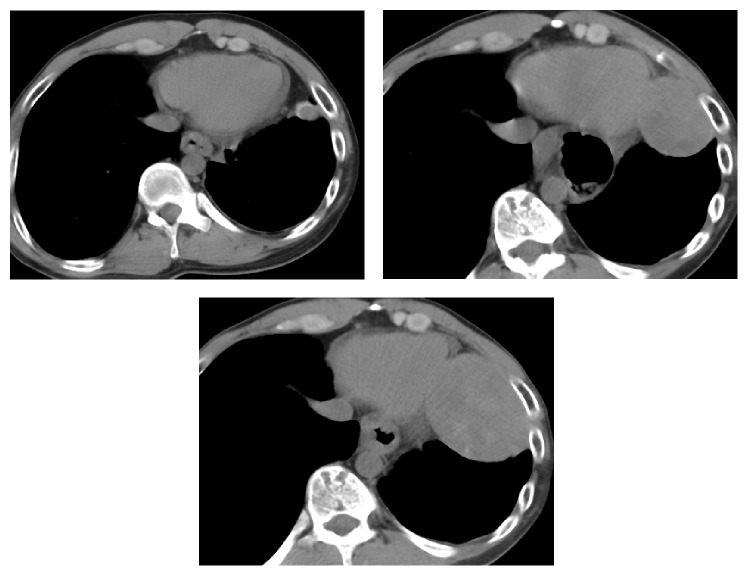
Noncontrast chest CT shows enlarging heterogeneous, well defined mass lesion at the level of left cardiophrenic recess (consecutive CTs from 2006, 2011, and 2013). The patient also had a hiatal hernia characterized by dilation and wall thickening of the distal esophagus. This was treated with Nissen fundoplication in 2012.
